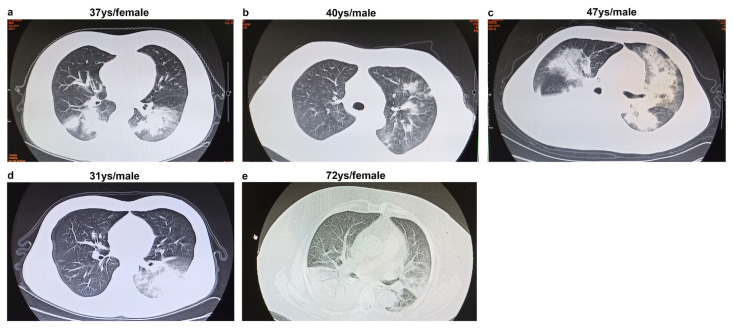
(a–e) Computed tomography images of five patients with adenoviral pneumonia showing consolidation and surrounding ground-grass opacity.
